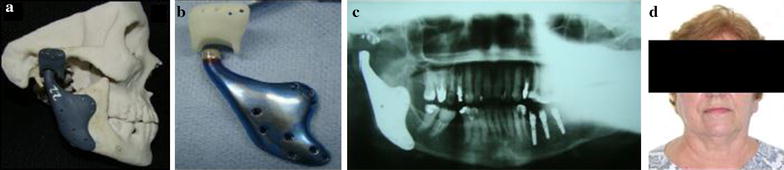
a Prototyping with the adapted TMJ prostheses; b TMJ prostheses image; c panoramic X-ray 2-year control; d patient image 2 years after surgery
